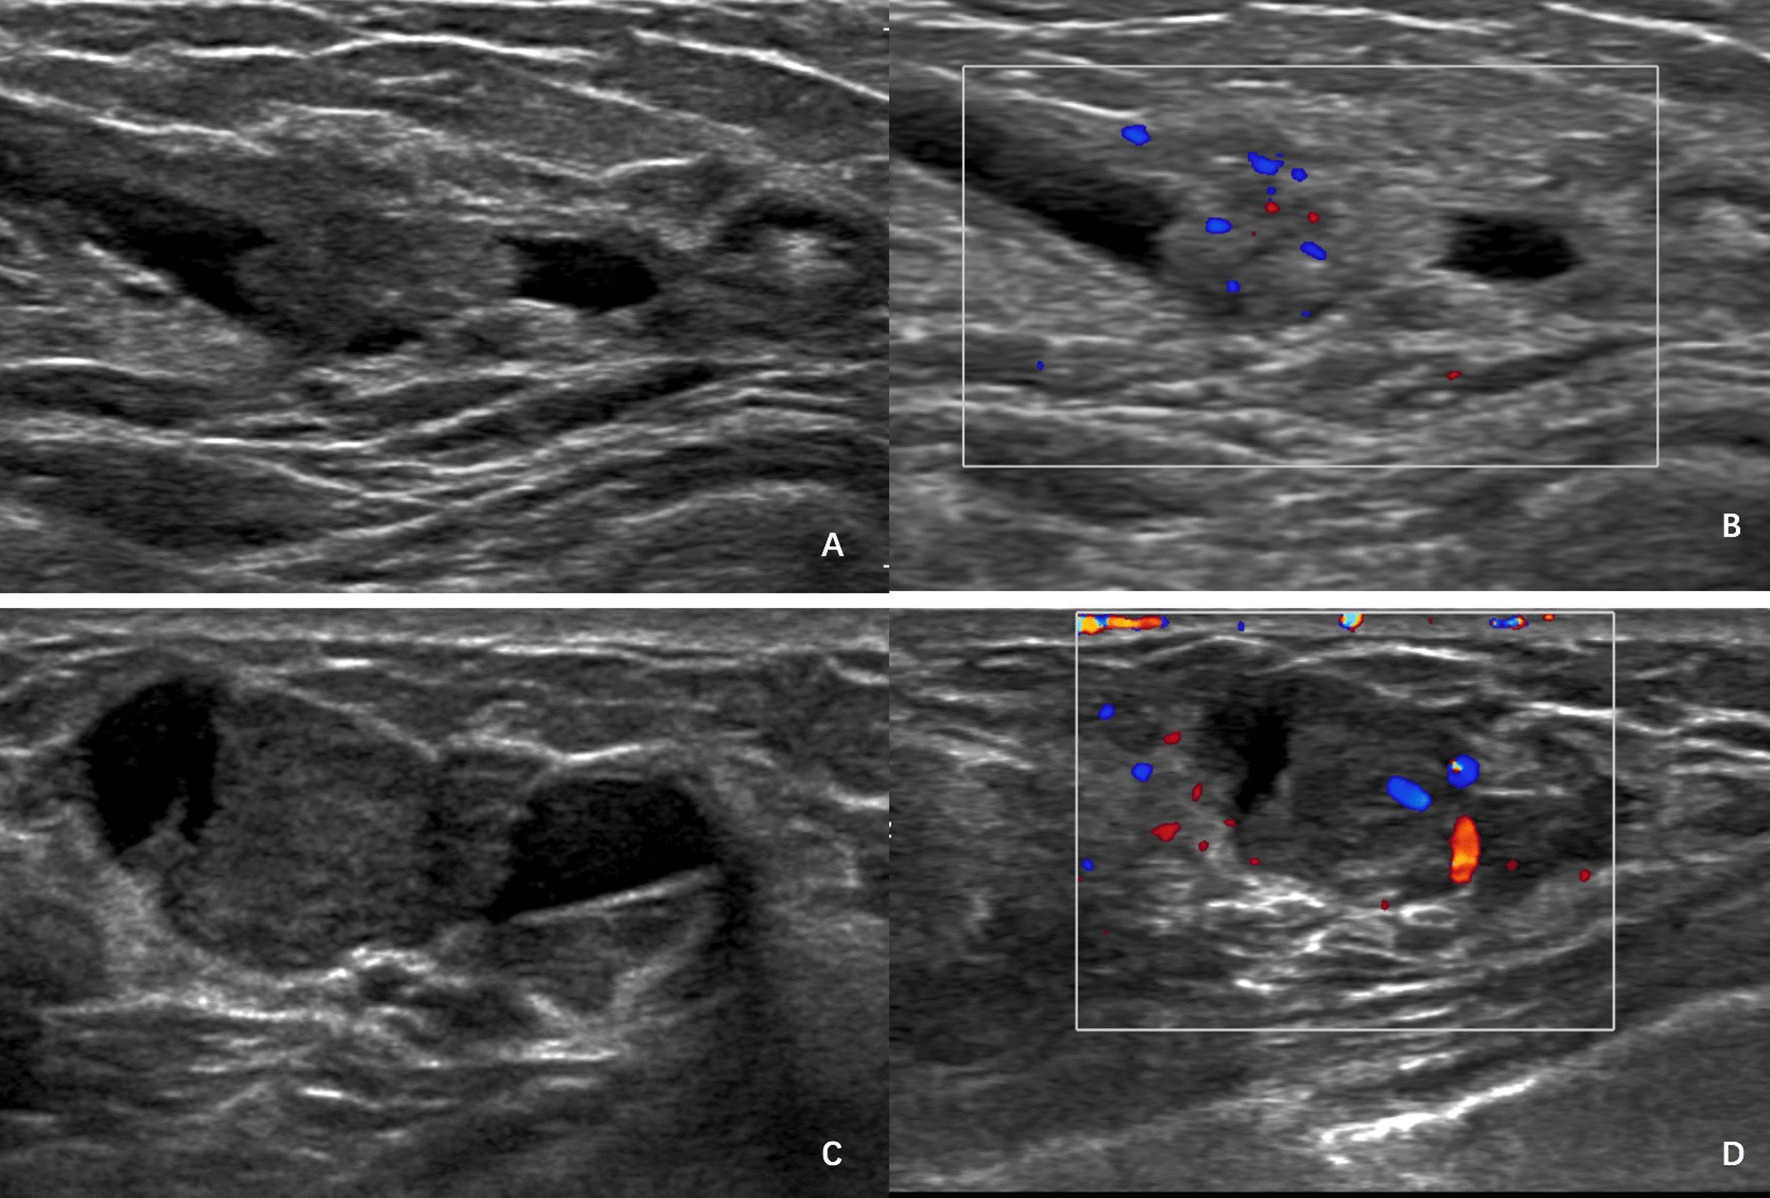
A breast masse from a young woman with intraductal papilloma. B-mode ultrasound showed a 2.0-cm narrow-base hypoechoic mass. The colour Doppler ultrasound image of the same mass reveals internal and peripheral blood flow signals (A,B). A breast masse from a young woman with intraductal papillary carcinoma, B-mode ultrasound showed a 0.9-cm wide-base hypoechoic mass. The colour Doppler ultrasound image of the same mass reveals internal and peripheral blood flow signals (C,D)
